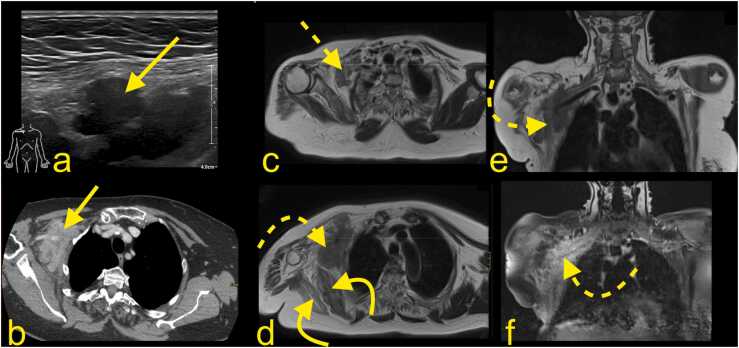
A 78-year-old patient underwent a mastectomy and partial axillary evacuation 15 years ago. Now, 1–2 months ago, she reported a swollen arm after trauma. The radiography showed no fracture. Ultrasound (a) revealed a mass in the right axillary cavity (arrow on a-e). (b) No thrombus in the axillary vessels or metastasis in the lungs were revealed on thoracic CT with i.v. contrast. The mass in the right axillary cavity (straight arrow on a and b) was biopsied, and breast cancer metastasis was confirmed. Radiation was administered with good clinical response (dashed arrow on c). After two years without symptoms, gradual clinical progress, and increasing weakness in the right arm, there was a suspicion of metastasis progress (d, e and f). Repeated MRI of the BP with (d) T2-weighted axial imaging, (e) T1-weighted TSE, and (f) T1-weighted imaging with i.v. contrast and fat suppression showed the metastasis (curved dashed arrow) with about 20% volume progress and infiltration of the right BP (dashed arrow). Muscle atrophy is seen on the right side (curved arrow).
